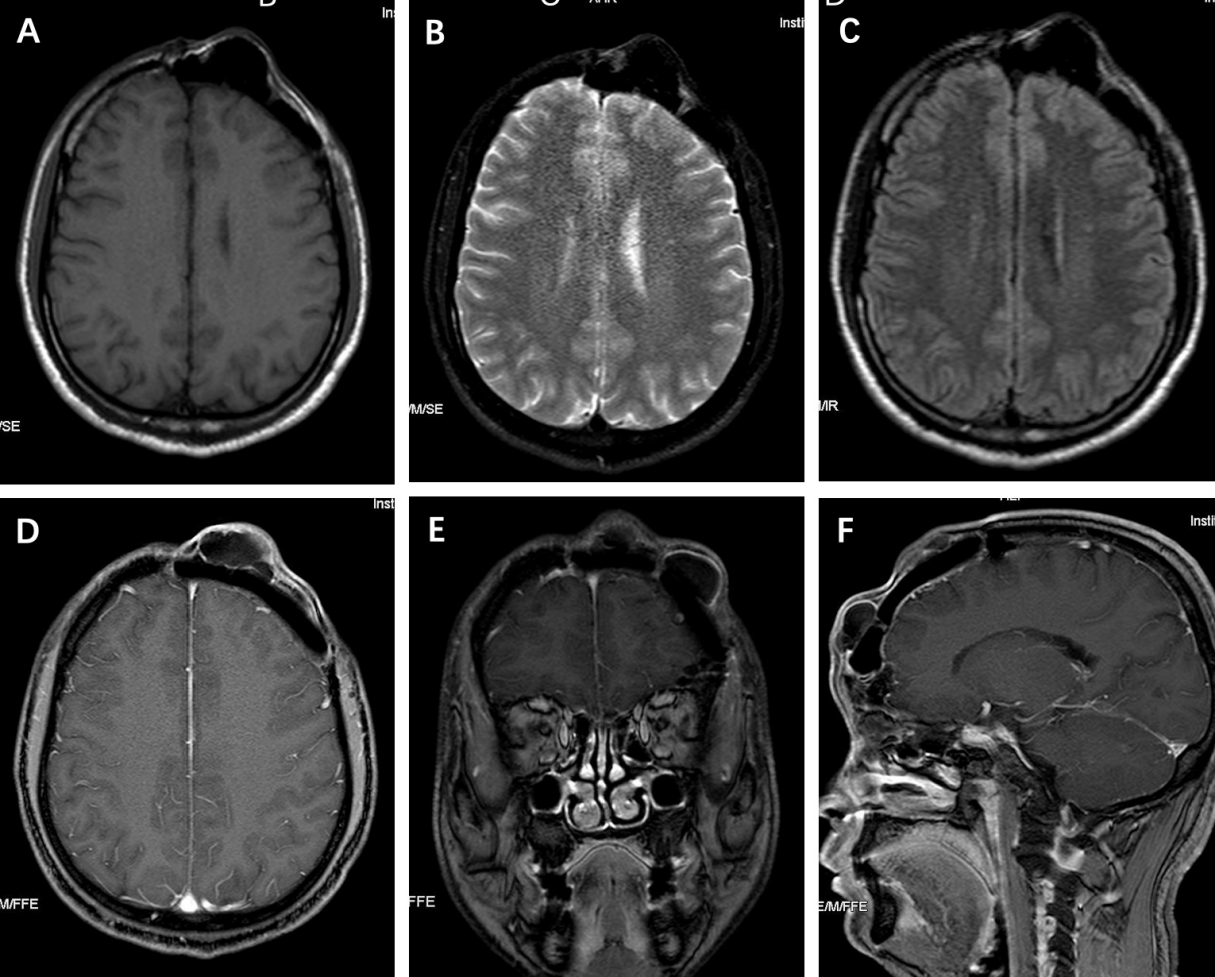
(A-F) All the MRI sequences depicted the lesions’ low signals.
